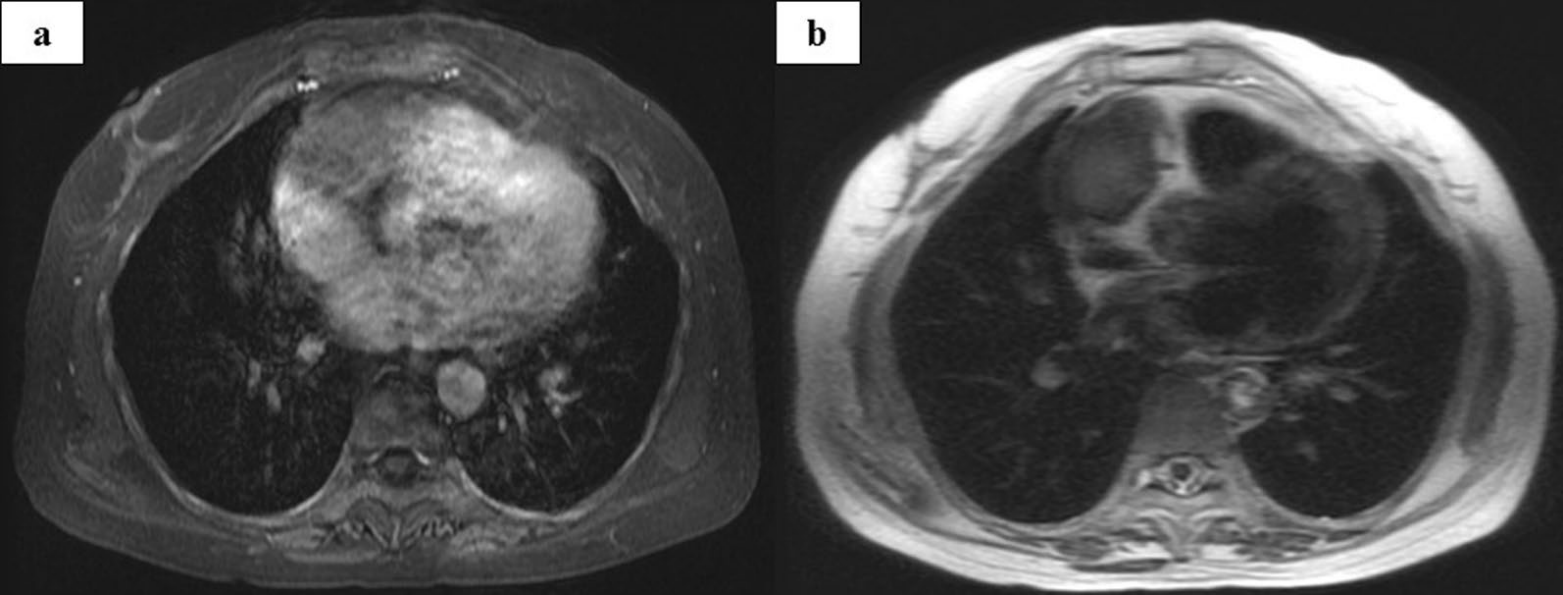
Chest magnetic resonance images showing a mass in the anterior mediastinum on a T1-weighted and b T2-weighted sequences
